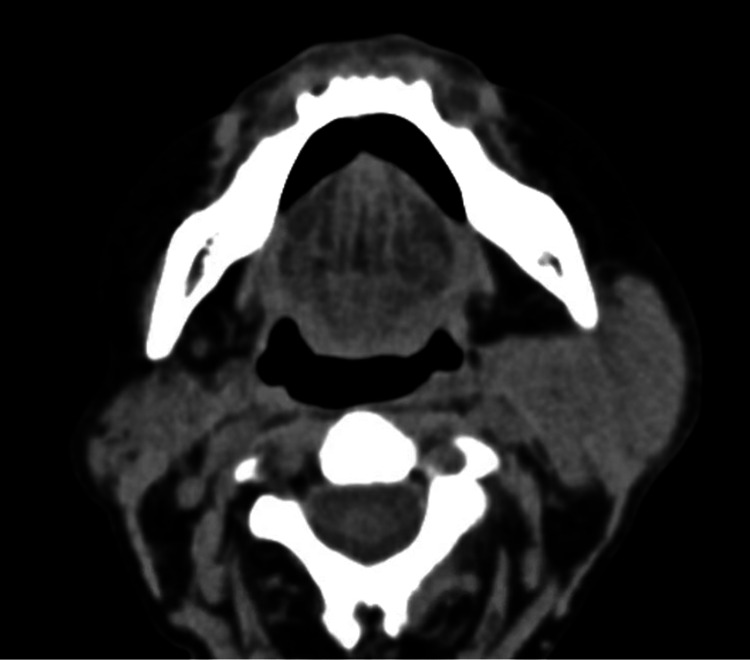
CT scan showing left parotid enlargement
